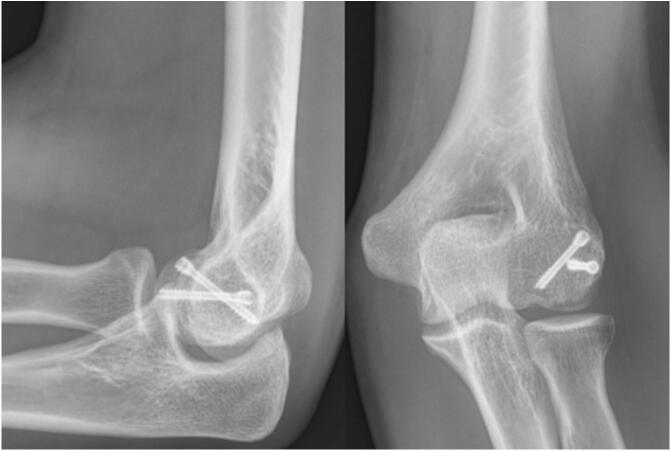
Elbow radiographs of patient 2 at 2-year follow-up.
